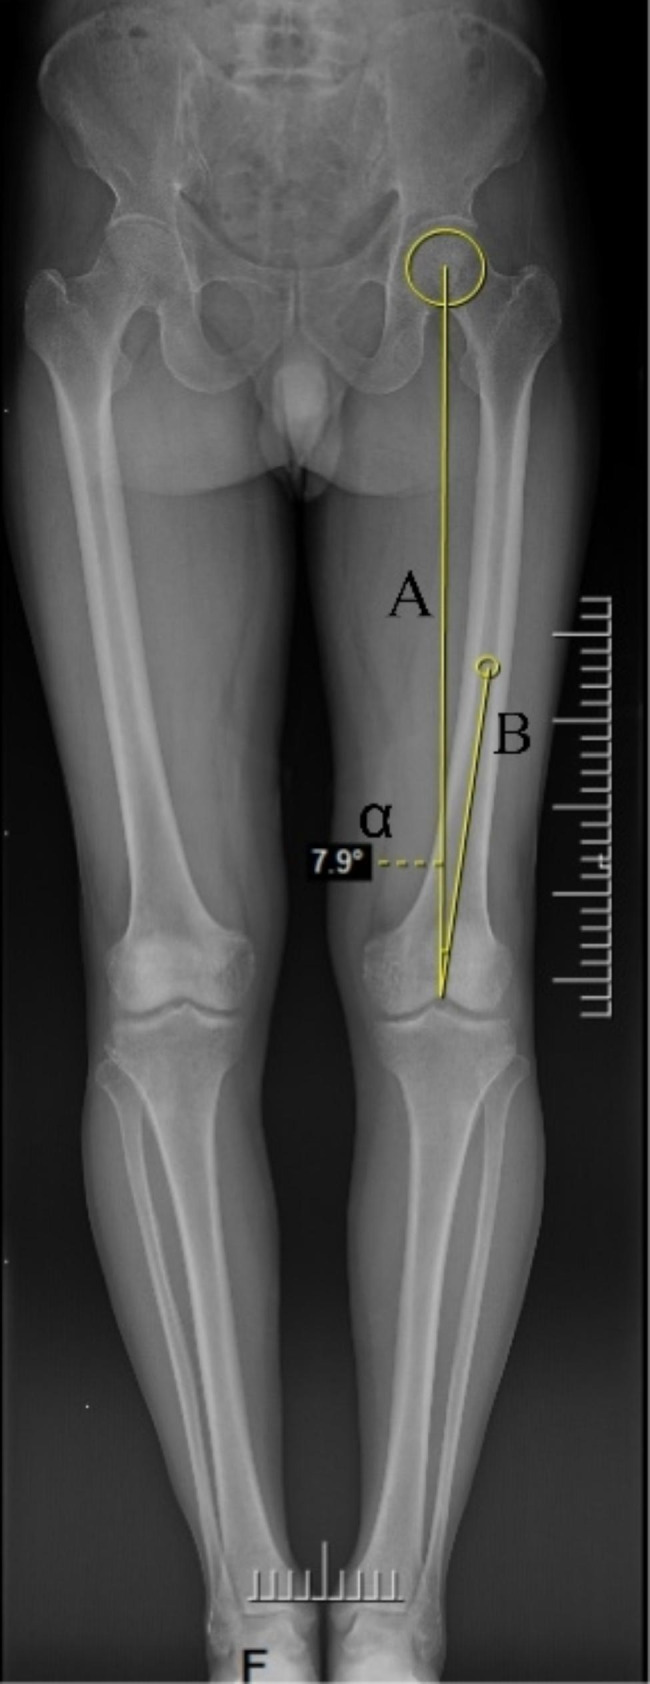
Measurement of femoral valgus angle in full-length radiographs of both lower limbs. Note: A: mechanical axis of the femur. B: anatomical axis of the femur. α: femoral valgus angle
